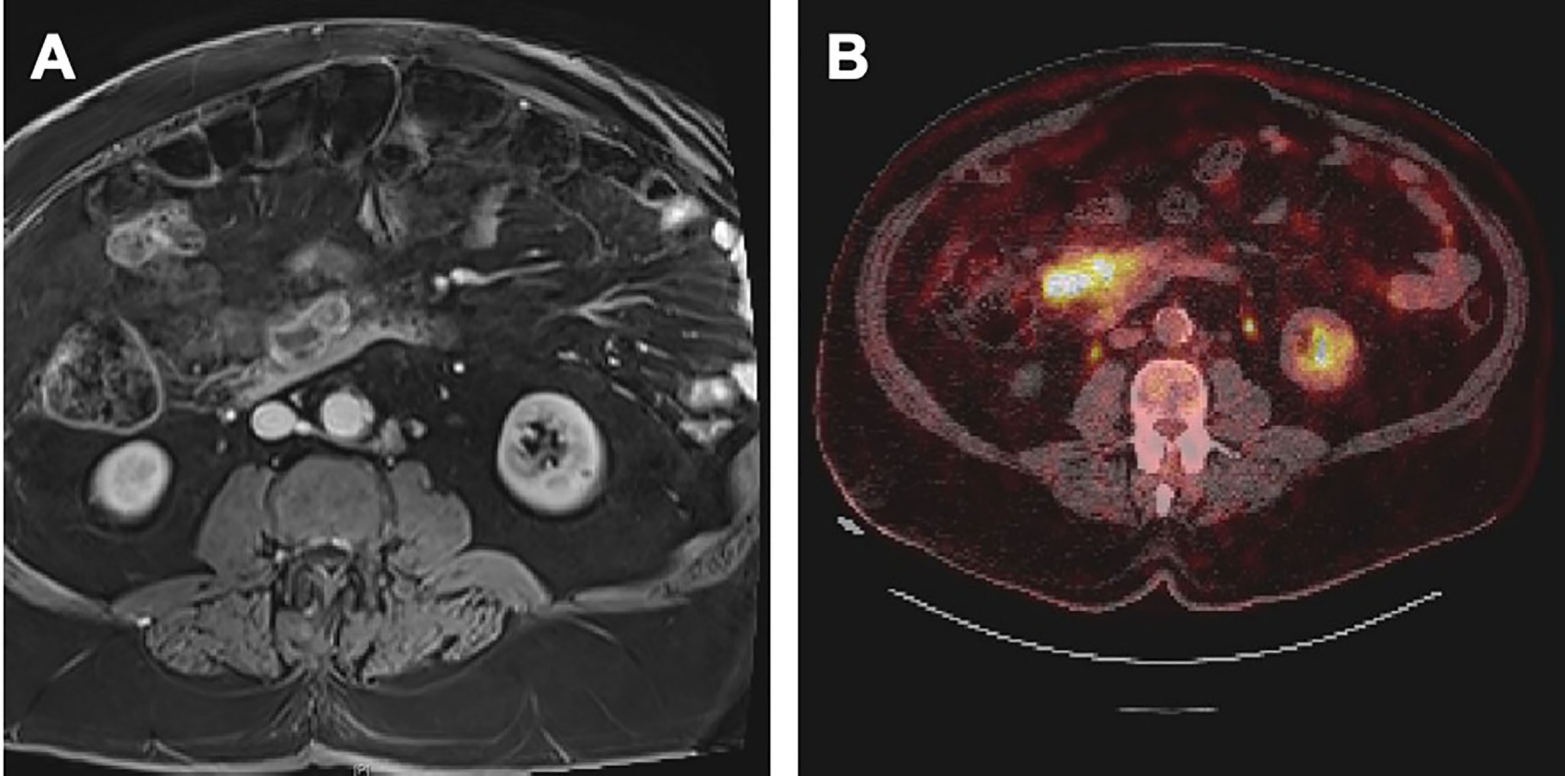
(A) MRI at the diagnosis. (B) PET/CT at the diagnosis.
